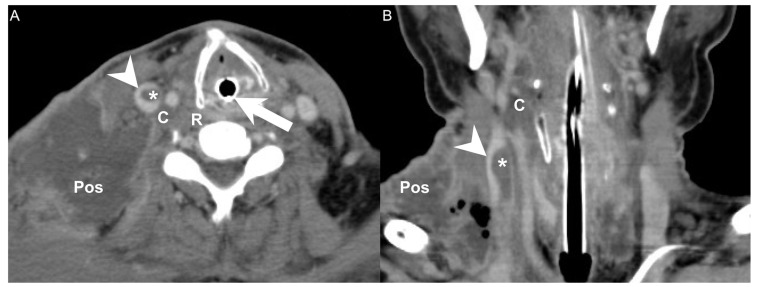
(A) CT axial view and (B) coronal view of a patient with concurrent DNI and LS. Arrowhead: internal jugular vein; Asterisk: thrombosis; Arrow: endotracheal tube; C: carotid space; Pos: posterior cervical space; R: retropharyngeal space.
